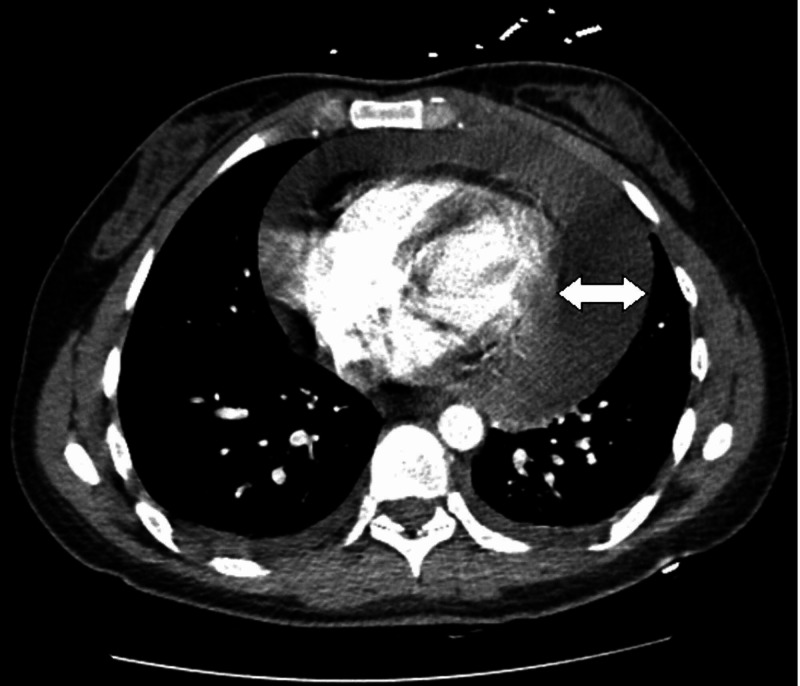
Axial view of the computed tomography angiogram of the chest revealing a large circumferential pericardial effusion
